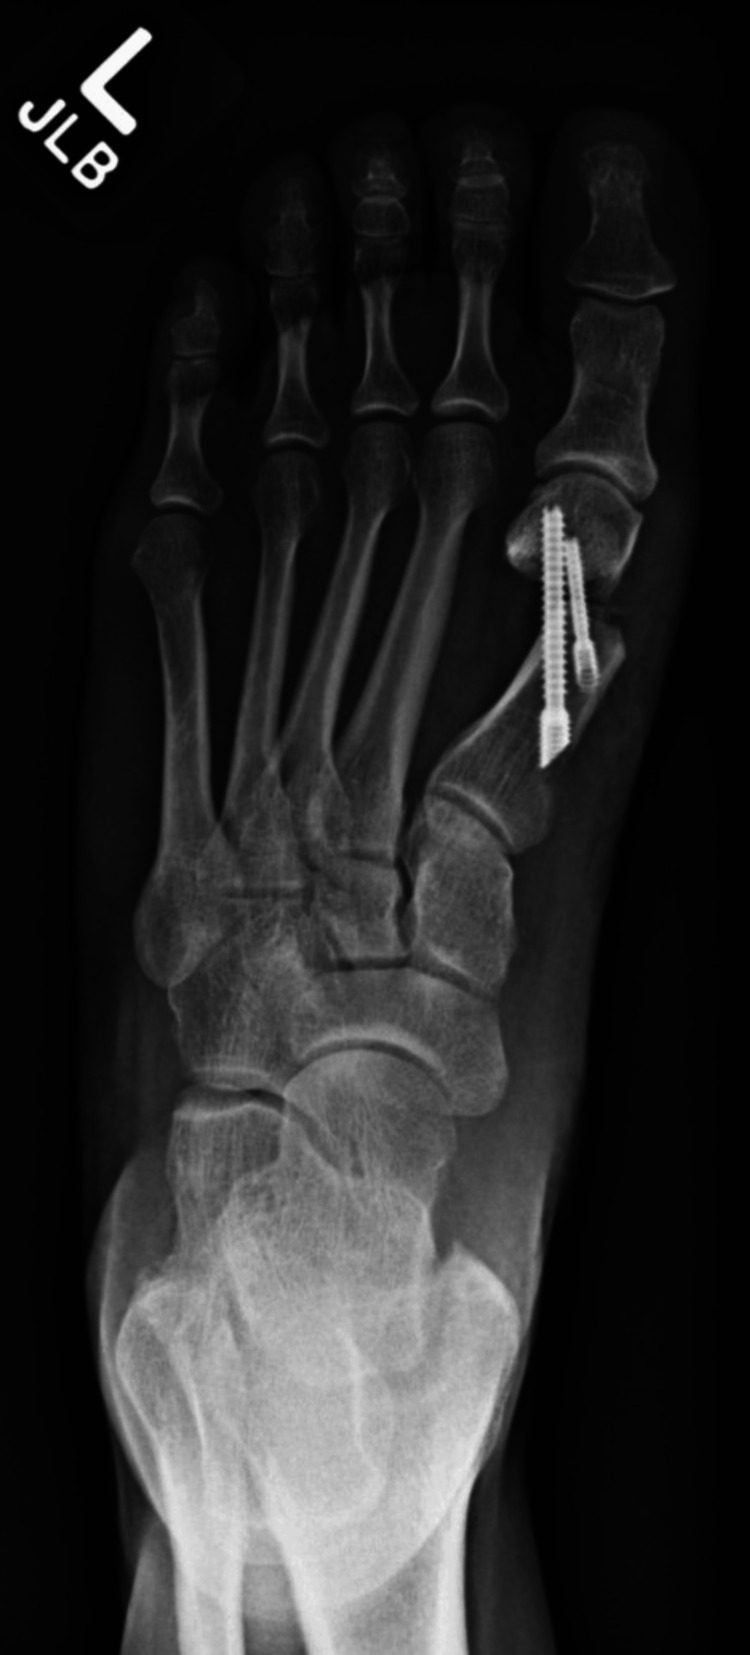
Radiograph two weeks after minimally invasive bunionectomy showing satisfactory alignment and hardware placement
